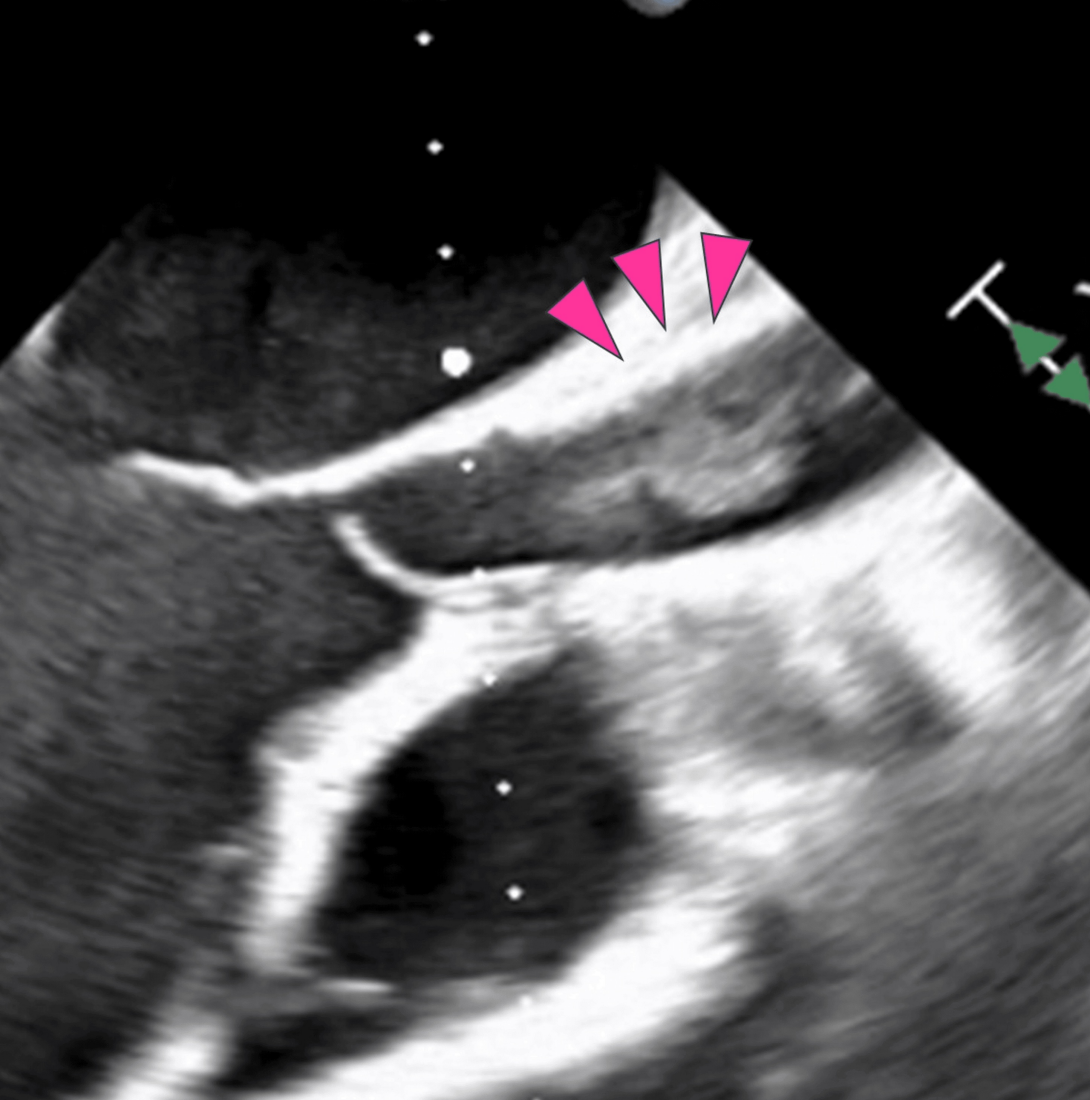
Transesophageal echocardiography A mobile thrombus was observed in the ascending aorta after the removal of Impella 5.5*. *Abiomed, Inc., Danvers, Massachusetts, United States
